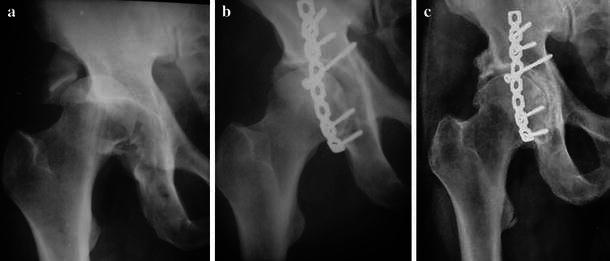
a Preoperative anteroposterior (AP) radiographs of a 42-year-old man showing posterior acetabular-wall fracture and associated hip-joint dislocation, b postoperative AP radiograph showing anatomical reduction, c AP radiographs at 20 years’ follow-up showing minimal sclerosis with mild osteoarthritic changes
